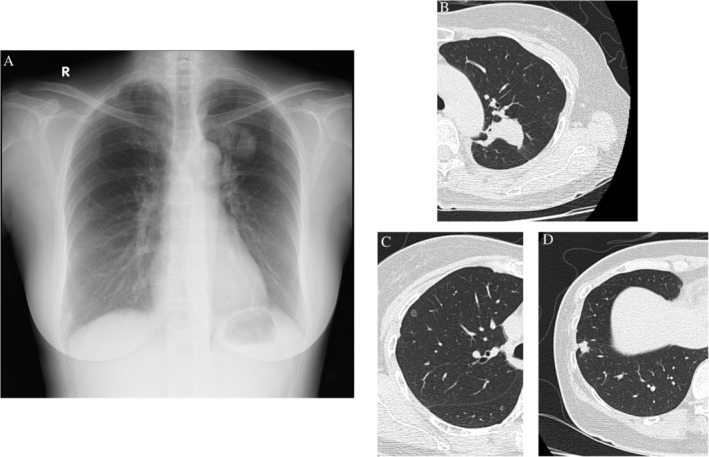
Chest X‐ray and computed tomography (CT). A chest X‐ray revealed a 4‐cm diameter mass in the left upper field. B chest CT revealed a 47‐mm diameter mass in the left segment (S) 1 + 2, C a 6‐mm diameter pure ground‐glass opacity in the right S3, and D an 11‐mm diameter solid nodule in the right S9.
